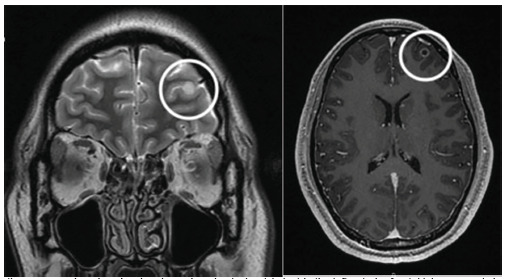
Magnetic resonance imaging showing ring enhancing lesion (circles) in the left anterior frontal lobe; coronal view at left and axial at right.
